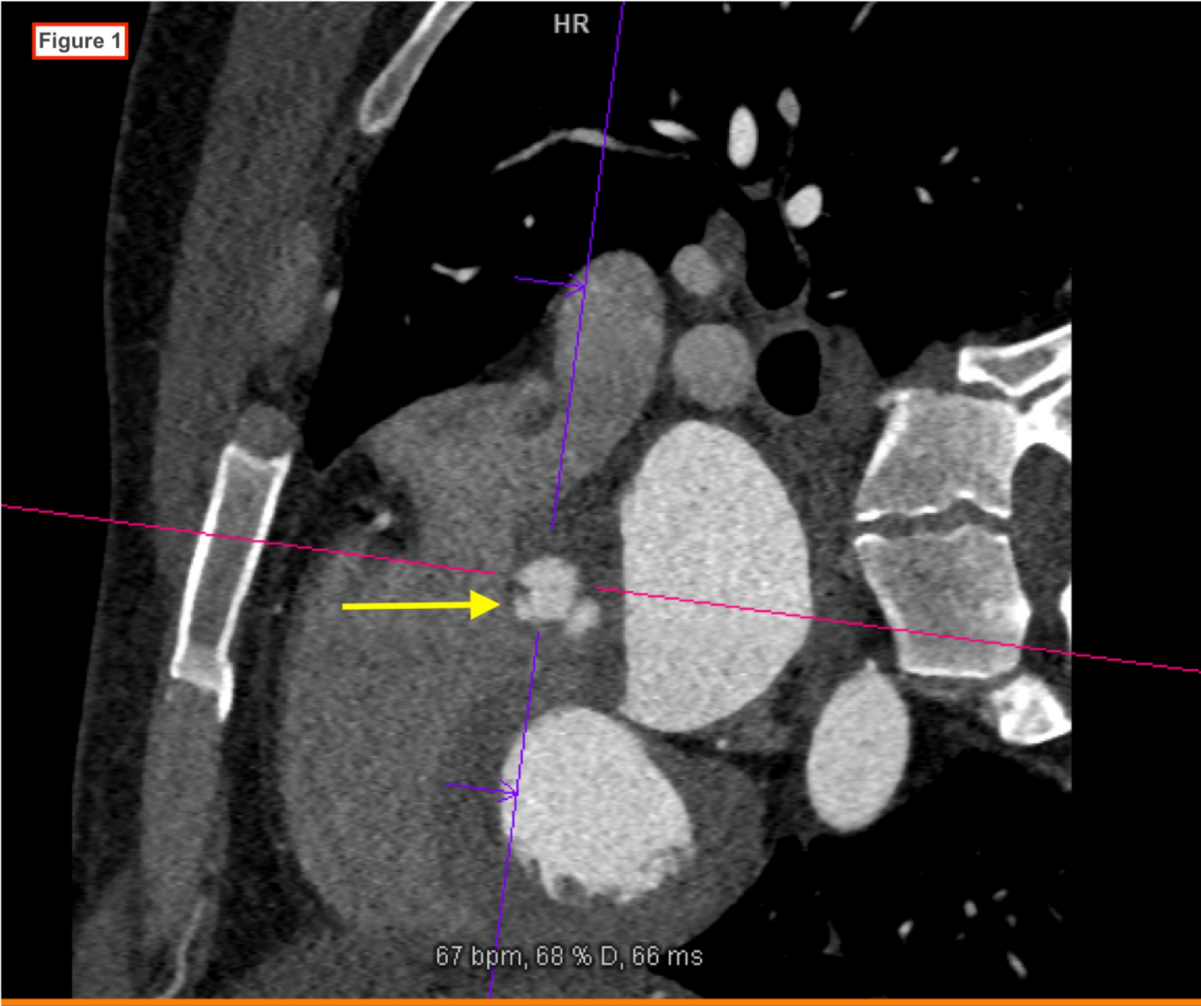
Cardiac CT image showing a 3 x 5 mm vegetation on the non-coronary leaflet of the aortic valve.
